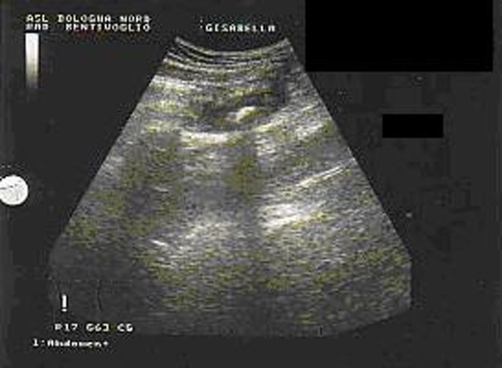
Abdominal ultrasonography showing an oval hypoechoic formation arising from the base of the cecum and the hyperechoic formation in it.
